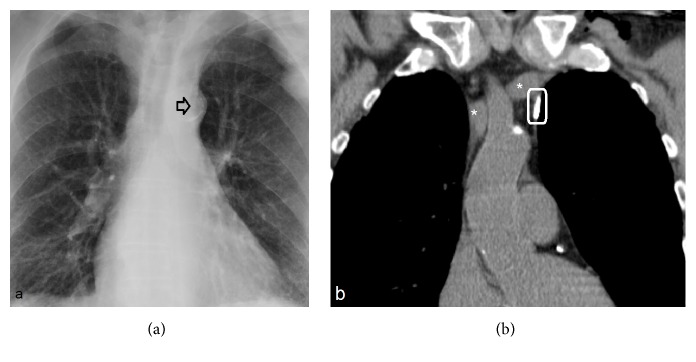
(a) The a.p. chest X-ray shows the catheter tip (black arrow) at the left margin of the mediastinum. (b) depicts a CT of the thorax. The catheter tip is marked in white. ^*∗*^left brachiocephalic vein.
